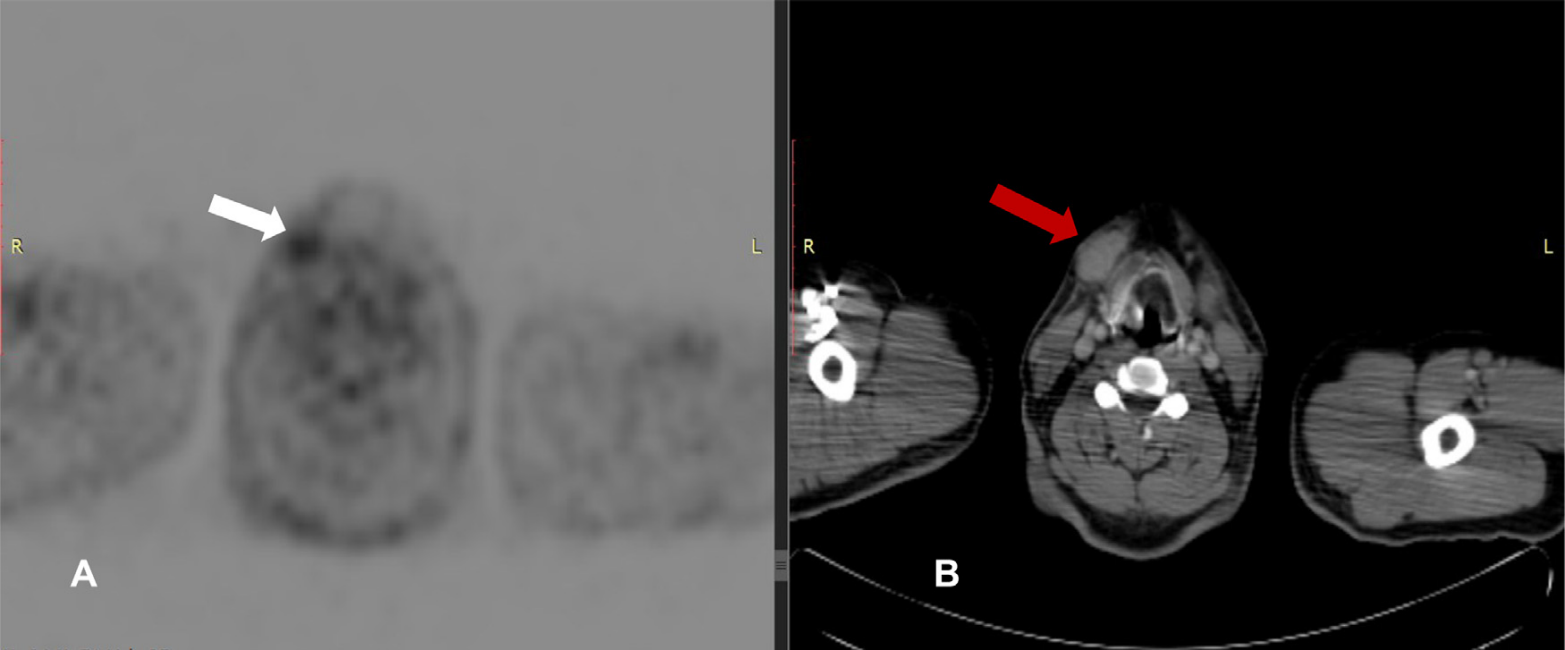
(A) Axial attenuation correction FDG PET image at the level of submandibular region shows a hypermetabolic lymph node SUV 2.3. (B) Axial CT component of the study at the same level reveals enlarged right submandibular lymph node.
